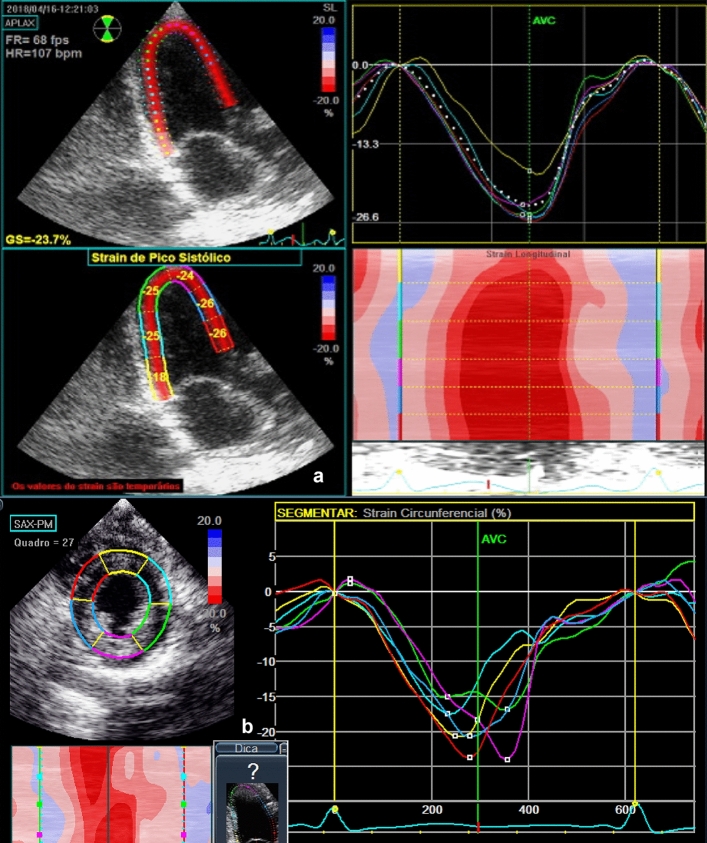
Two-dimensional speckle tracking echocardiogram. (a) Apical 3 chamber view, and (b) parastenal short axis view at the level of the papillary muscles.
